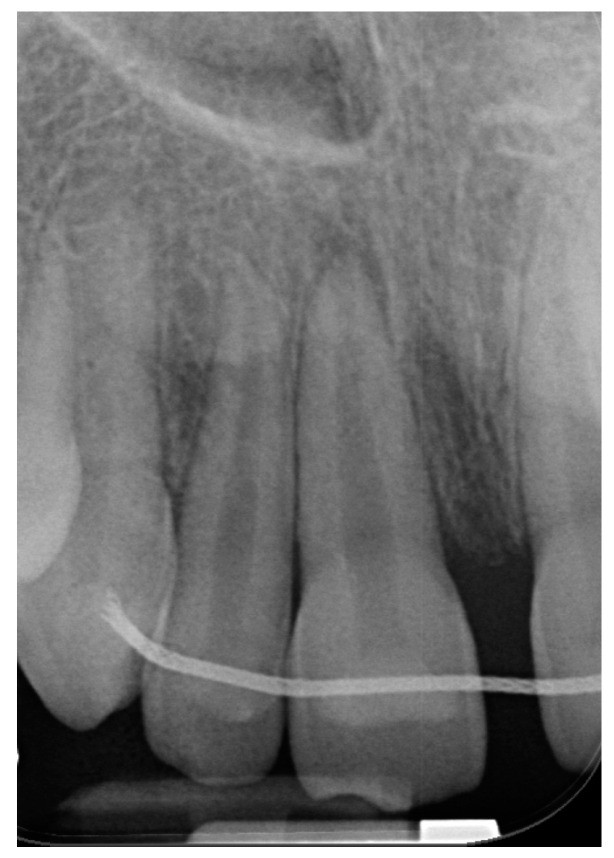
Case 2 radiograph post-replantation. Tooth #11 was re-implanted and fixated after evulsion.
